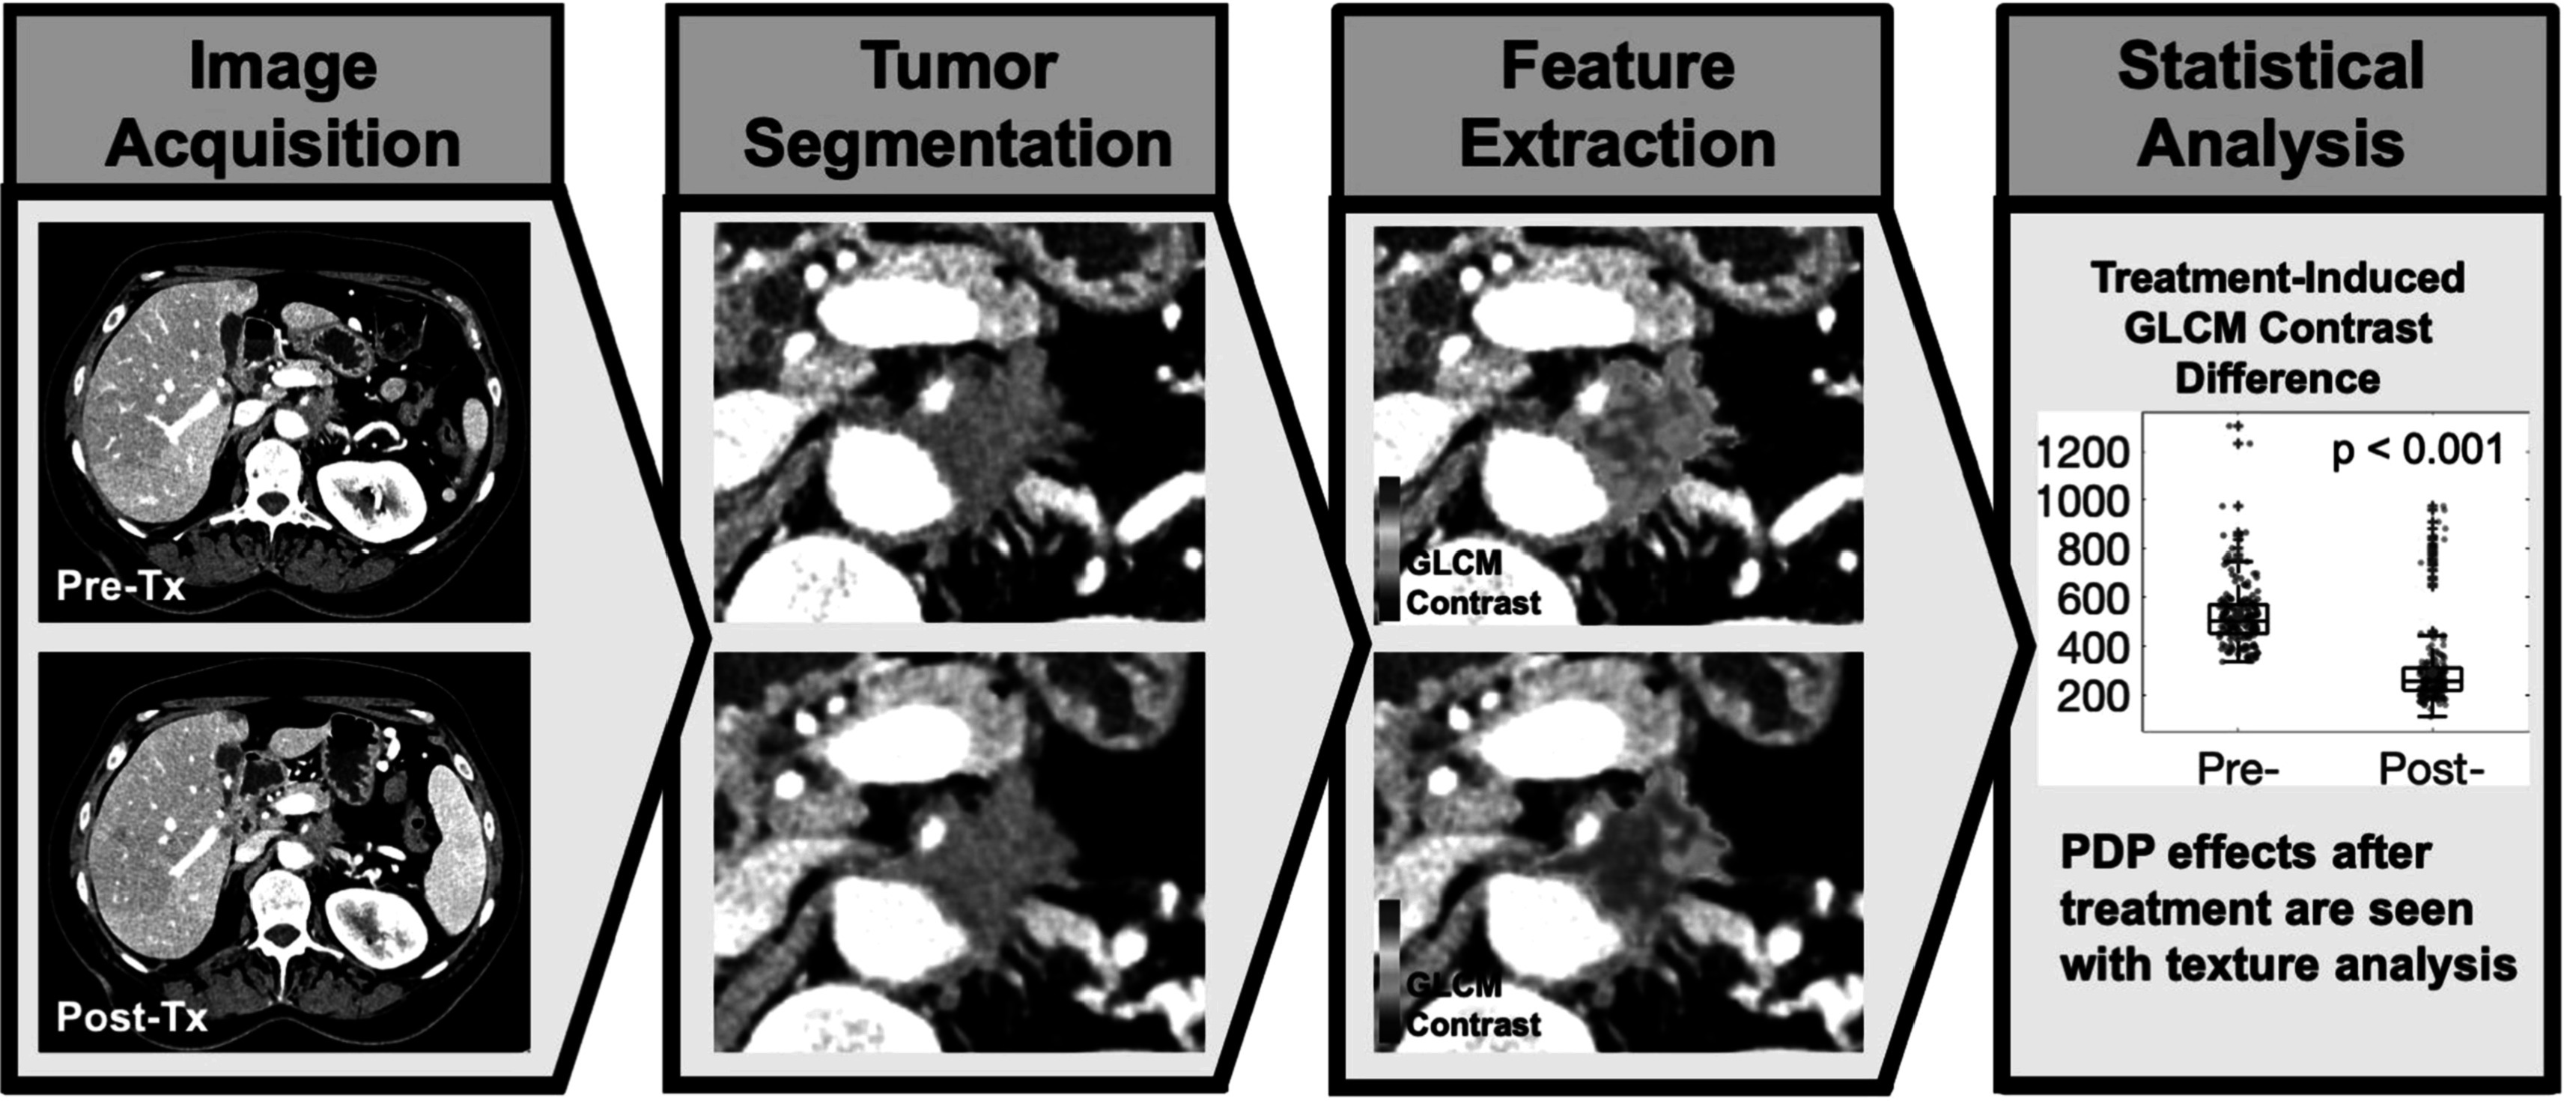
Overview of texture analysis in assessment of photodynamic therapy treatment on PDAC patients. Each patient received a pre-treatment and a post-treatment CT scan. Pancreatic tumor segmentation was performed, and texture analysis was carried out to extract underlying features that showed significant differences after the treatment.
